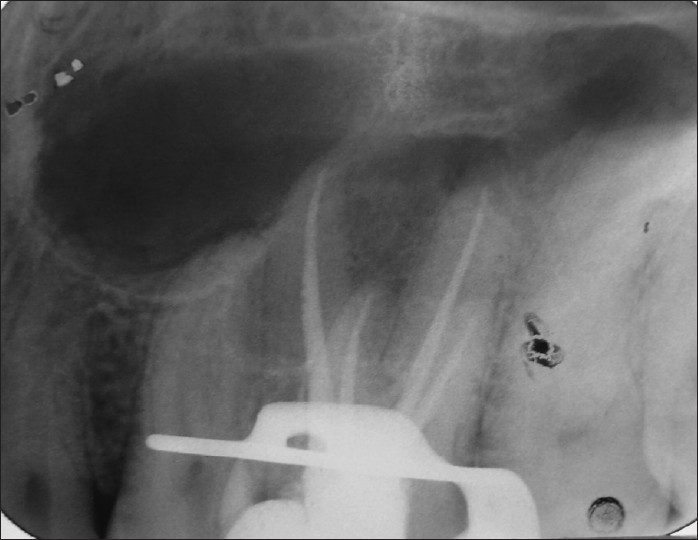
Post obturation radiograph of 26
